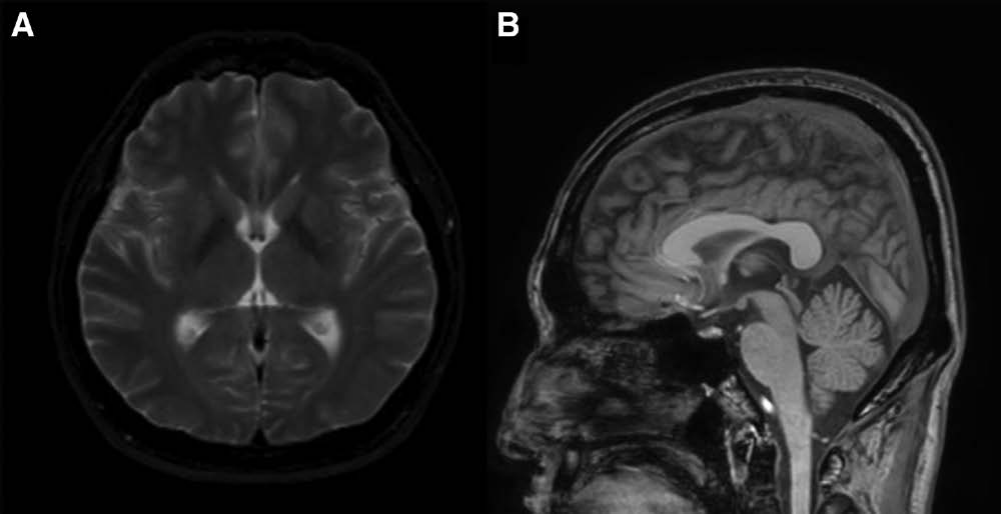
(A) Axial T2-weighted image. (B) Sagittal T1-weighted image. No abnormalities were observed on the patient’s brain MRI. MRI = magnetic resonance imaging.
